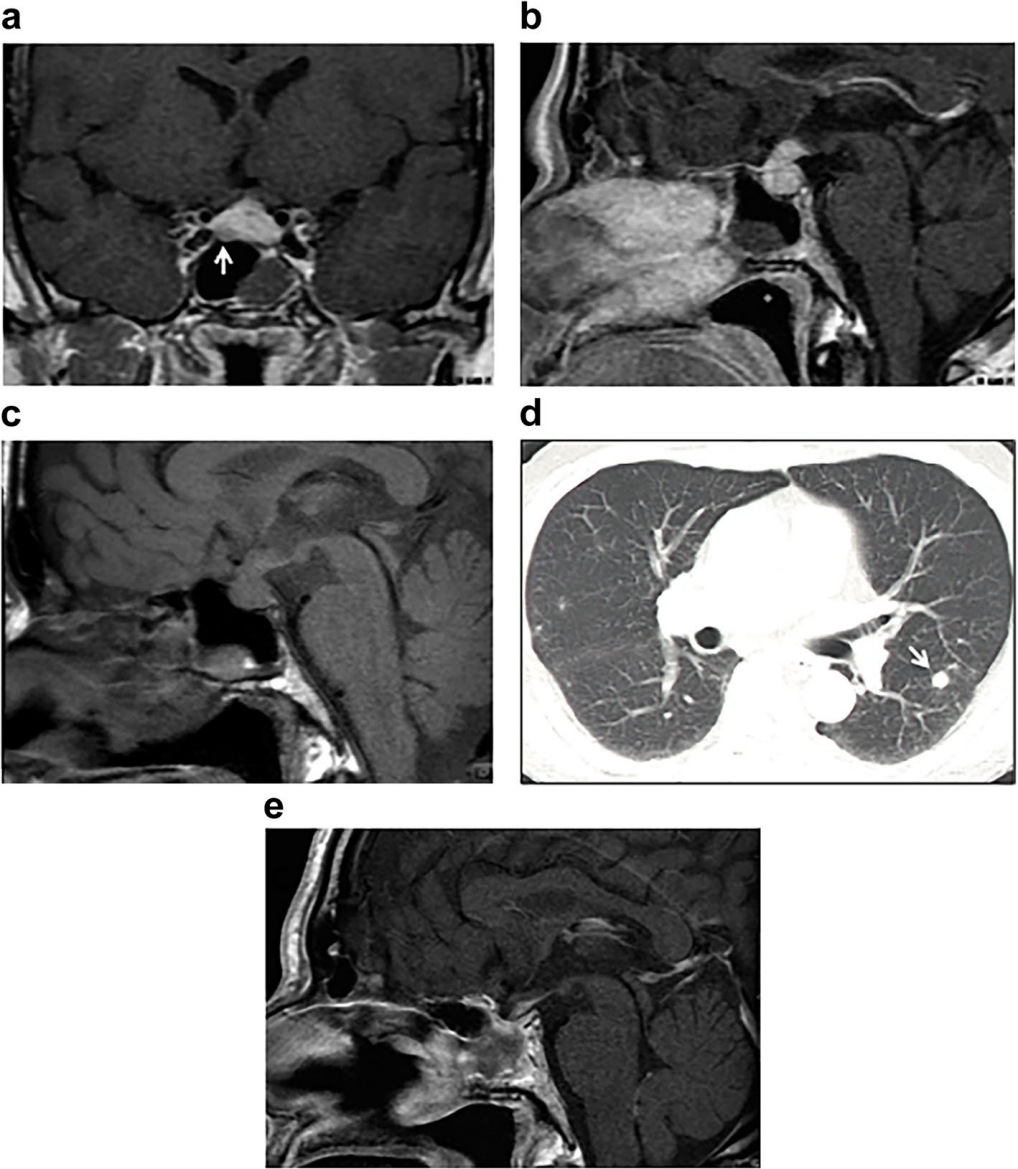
Magnetic resonance imaging (MRI) showing swelling of the pituitary body and stalk (a) and a 7-mm poorly enhanced mass in the right anterior lobe (b: arrow) before biopsy. On non-contrast MRI T1-weighted images, the high signal in the posterior pituitary lobes had disappeared (c). Chest computed tomography showed a nodular lesion in the left lung field (d: arrow). On MRI, the pituitary gland was found to be reduced in size after prednisolone treatment for 2 months (e)
